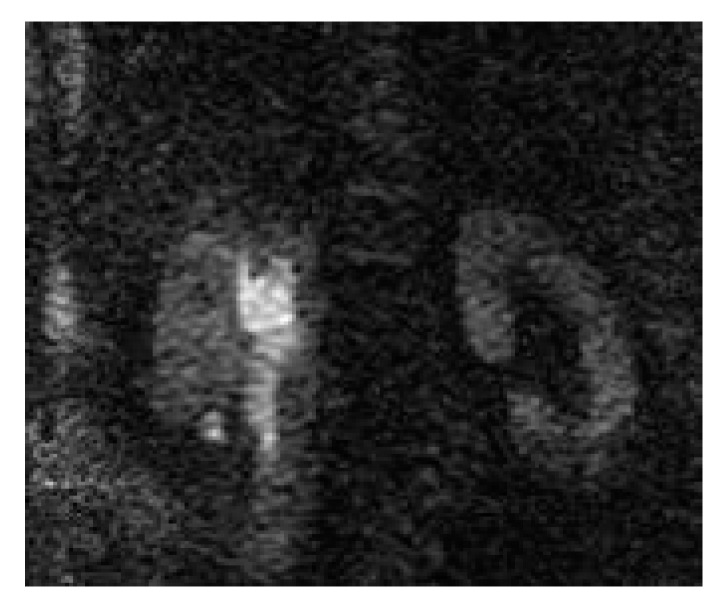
Diffusion-weighted image (DWI) of right renal and perirenal abscess with diffusion restriction.
